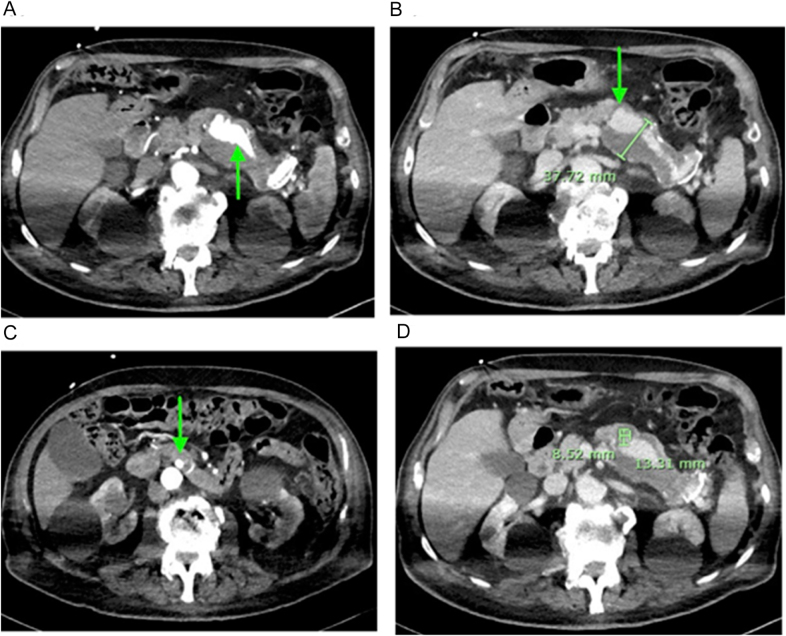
CTA abdomen and pelvis revealing an approximately 4.0 cm fusiform pseudoaneurysmal dilation of the splenic artery (a); an anteriorly projected 13 mm saccular component in the pseudoaneurysm body (b); an 8 mm pseudoaneurysm near the pancreatic uncinate process, likely of the pancreaticoduodenal artery (c); and the 8 mm pseudoaneurysm and 13 mm saccular components with official measurements (d).
